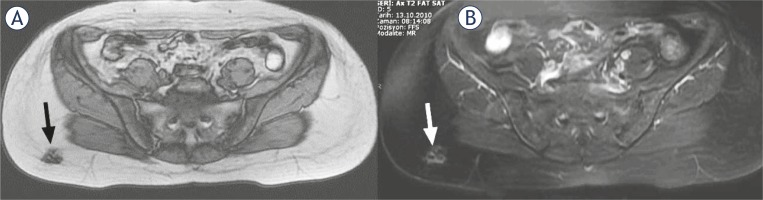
Axial magnetic resonance images. A: T1W image shows a hypointense lesion (black arrow) in adipose tissue in the postero-lateral part of right gluteal region. B: T2W fat-suppressed image shows hyperintense lesion (white arrow) with slightly irregular borders, internal structure slightly heterogeneous in the same region.
